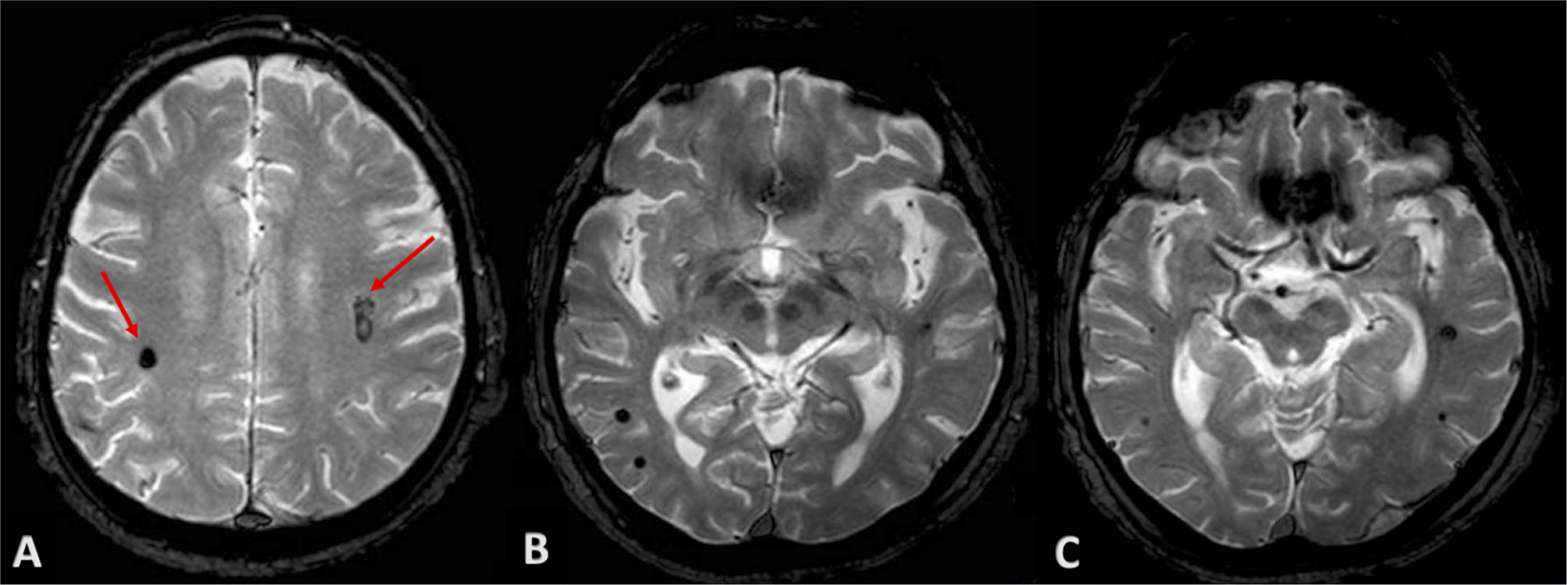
Patient's MRI at follow-up. Gradient-echo T2*-weighted sequences revealed roundish lesions underlying well-known intraparenchymal hemorrhages (A, red arrows), with classic magnetic resonance appearance of cavernous venous malformations. (B,C) show multiple and similar lesions throughout subcortical white matter and basal ganglia, bilaterally.
